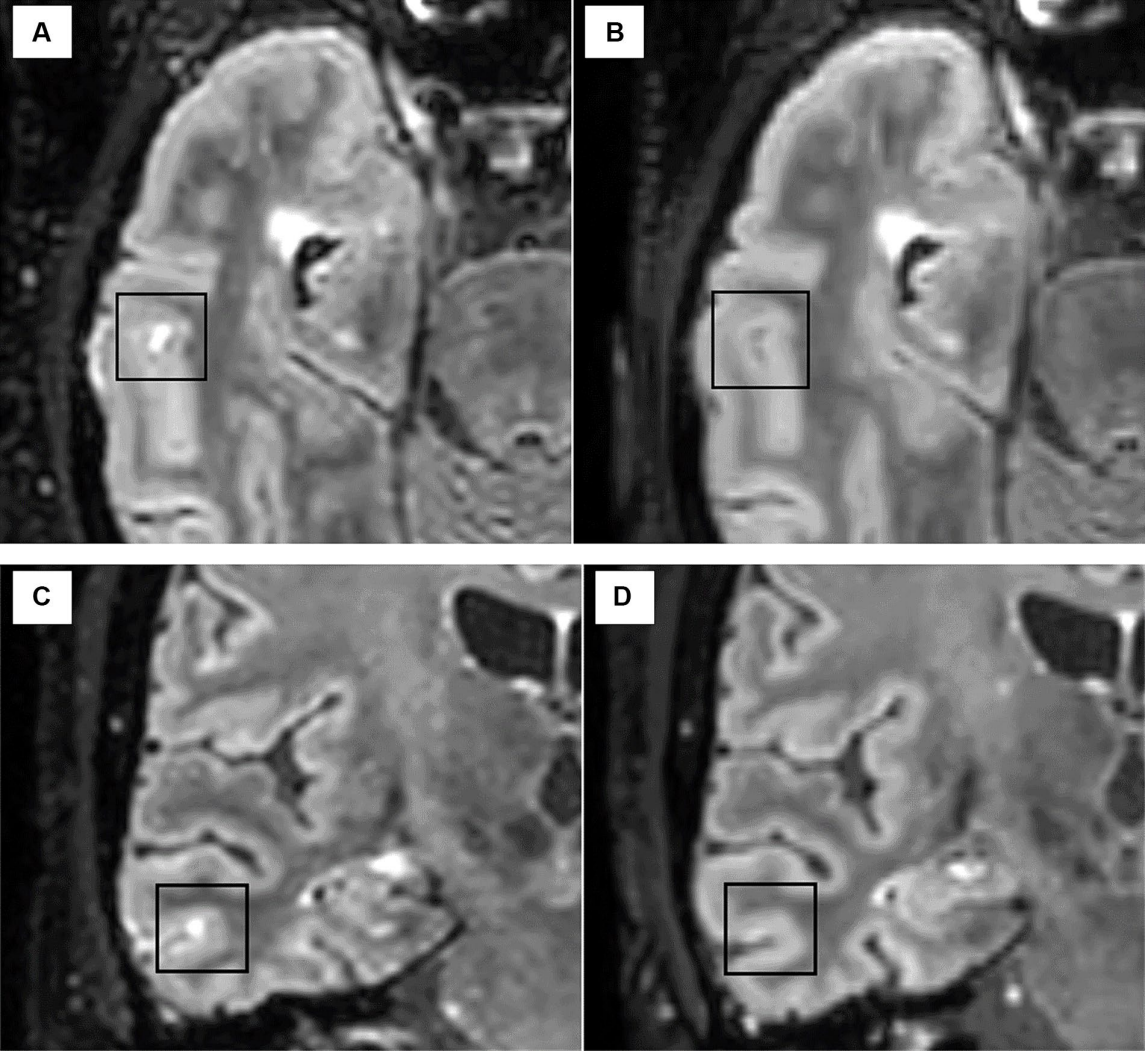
The disappearance of one LME focus was observed in one patient after AHSCT from the pre-treatment scan [axial (A) and coronal (C)] to the MRI scan taken at 6 months after AHSCT [axial (B) and coronal (D)].
